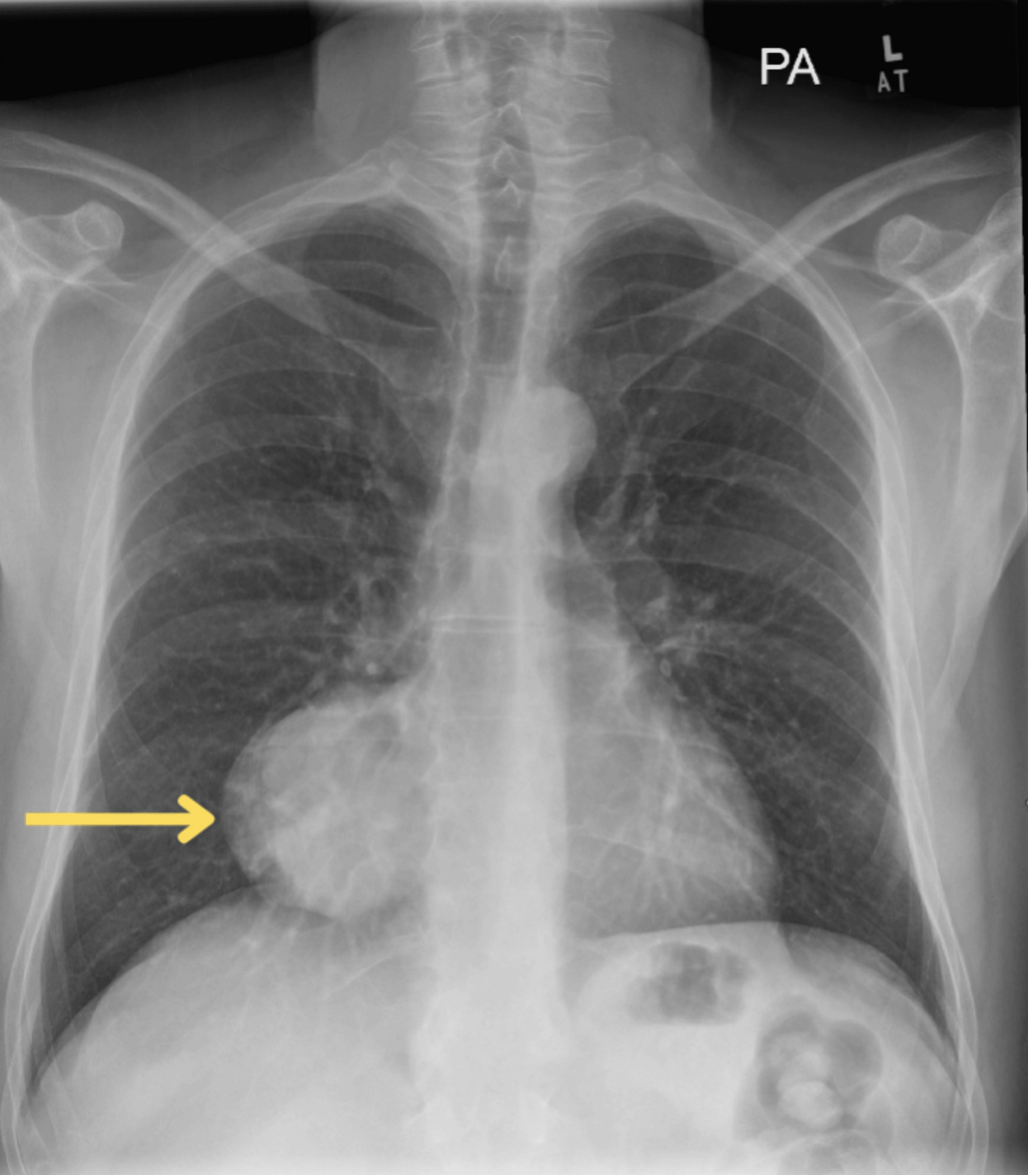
Chest X-ray showing a rounded opacity in the right lower zone, abutting the cardiac silhouette (yellow arrow)
